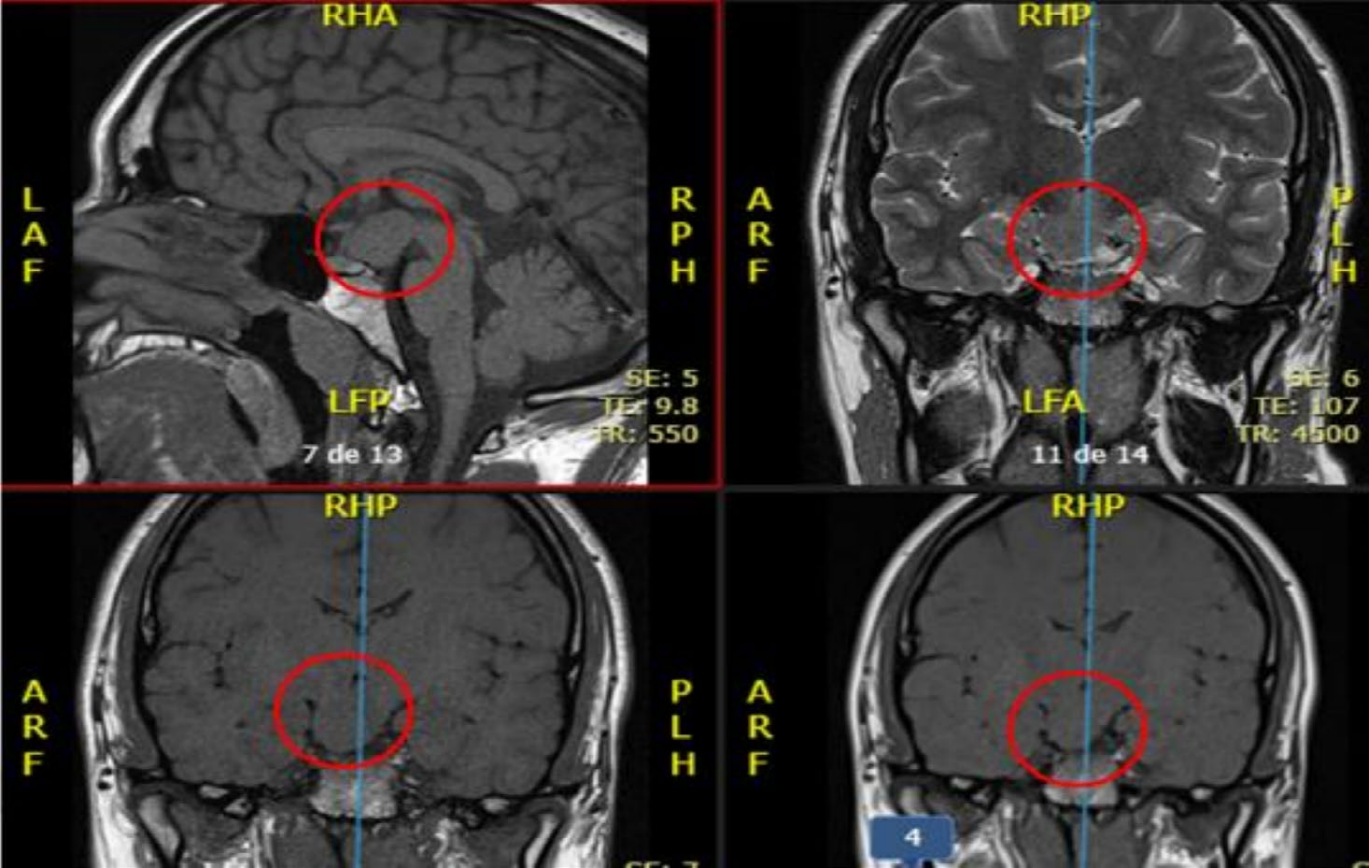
Magnetic resonance imaging (MRI) of the brain with sagittal and coronal views, performed at 22 years of age, showing a hypothalamic hamartoma centered on the tuber cinereum with cisternal and intraventricular involvement (red circle).
